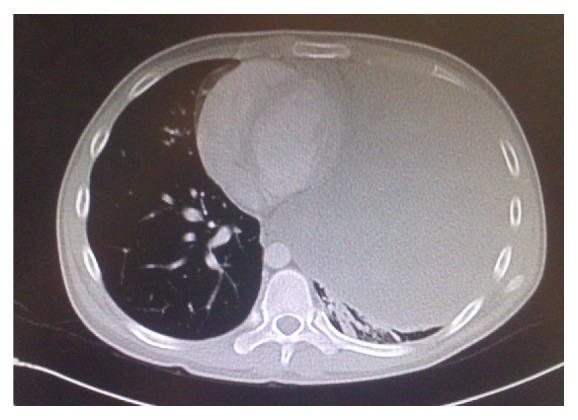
Anteroposterior view of CT scan showing that the mass occupies the whole of the left thoracic space.
